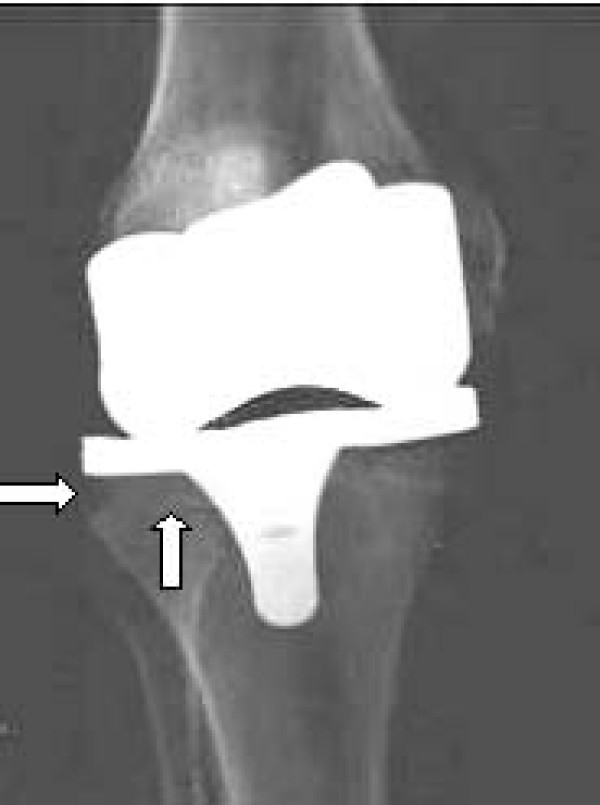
Antero-posterior plain X-ray of tibial osteolytic defect (large) as shown in Figure 1.
